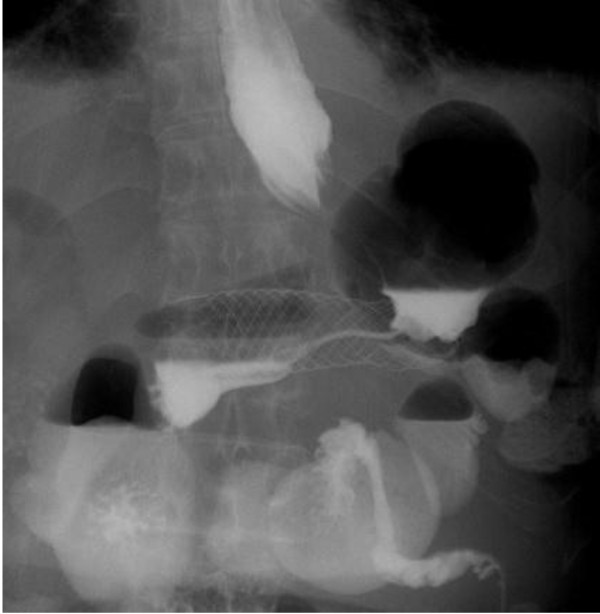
Contrast radiography on the day after stent placement. The self-expandable metal stent was fully expanded and the carcinomatous stricture of the gastric body was released, as seen on contrast radiography.
